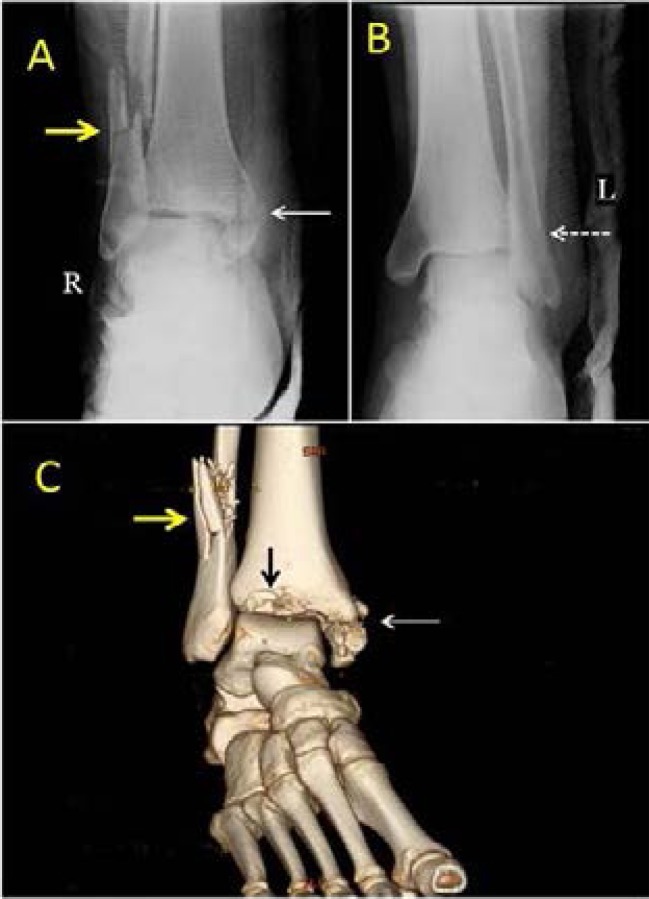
17-year-old male front seat passenger was involved in a front impact road traffic collision. The impact involved both feet. He sustained comminuted fracture of the right distal fibula (Yellow arrow), fracture of right medial malleolus (solid white arrow), and transverse fracture of the distal left fibula (white dashed arrow). CT scan with reconstruction was performed to demonstrate the behaviour of the fracture (C) showing a fracture of the anterior rim \of the distal tibia (black arrow) (Courtesy of Dr Ihab Abbas, Consultant orthopaedic Surgeon, Al Ain Hospital, Al Ain, UAE).
